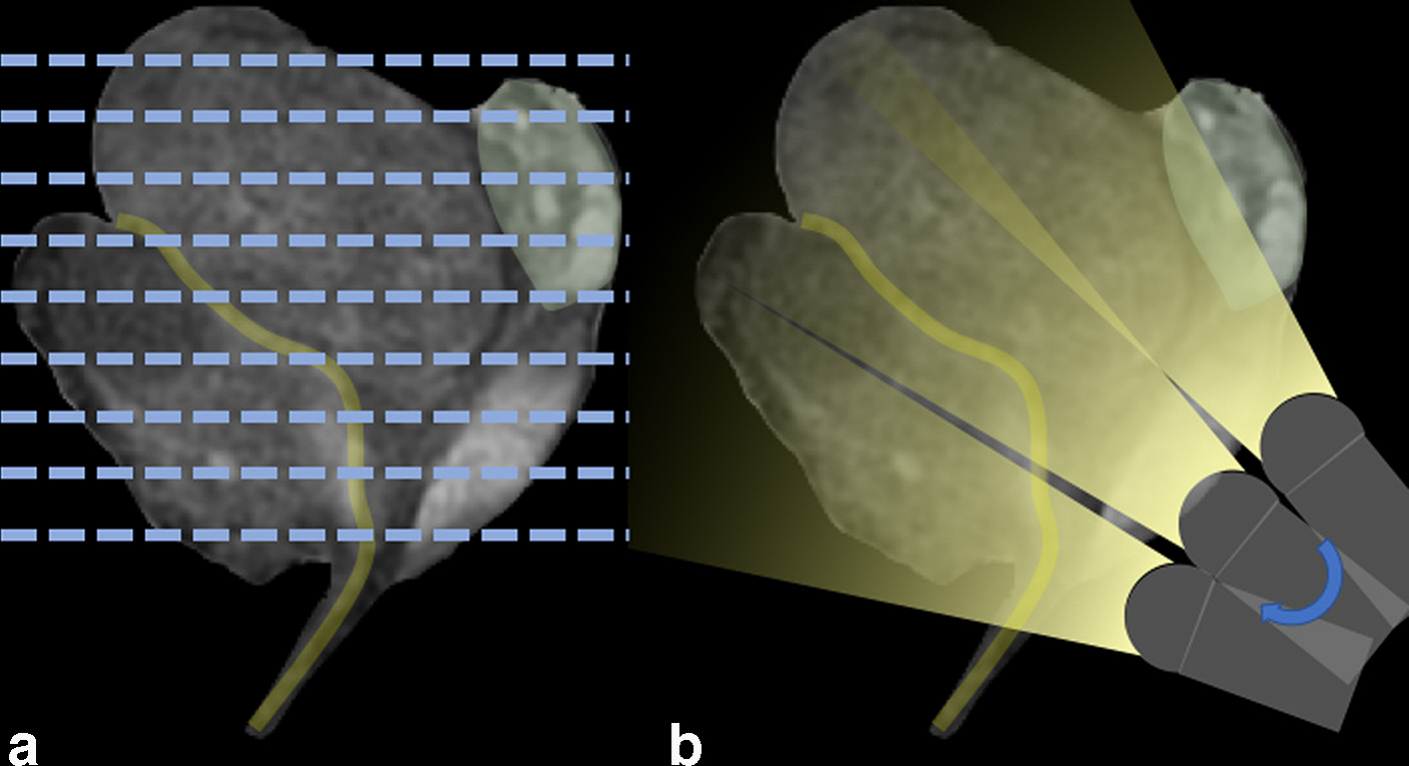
MRI and TRUS images are obtained with different obliquity, a factor that must be taken into consideration when considering the reported location of lesions during biopsies performed under cognitive co-registration. TRUS,transrectal ultrasound.
